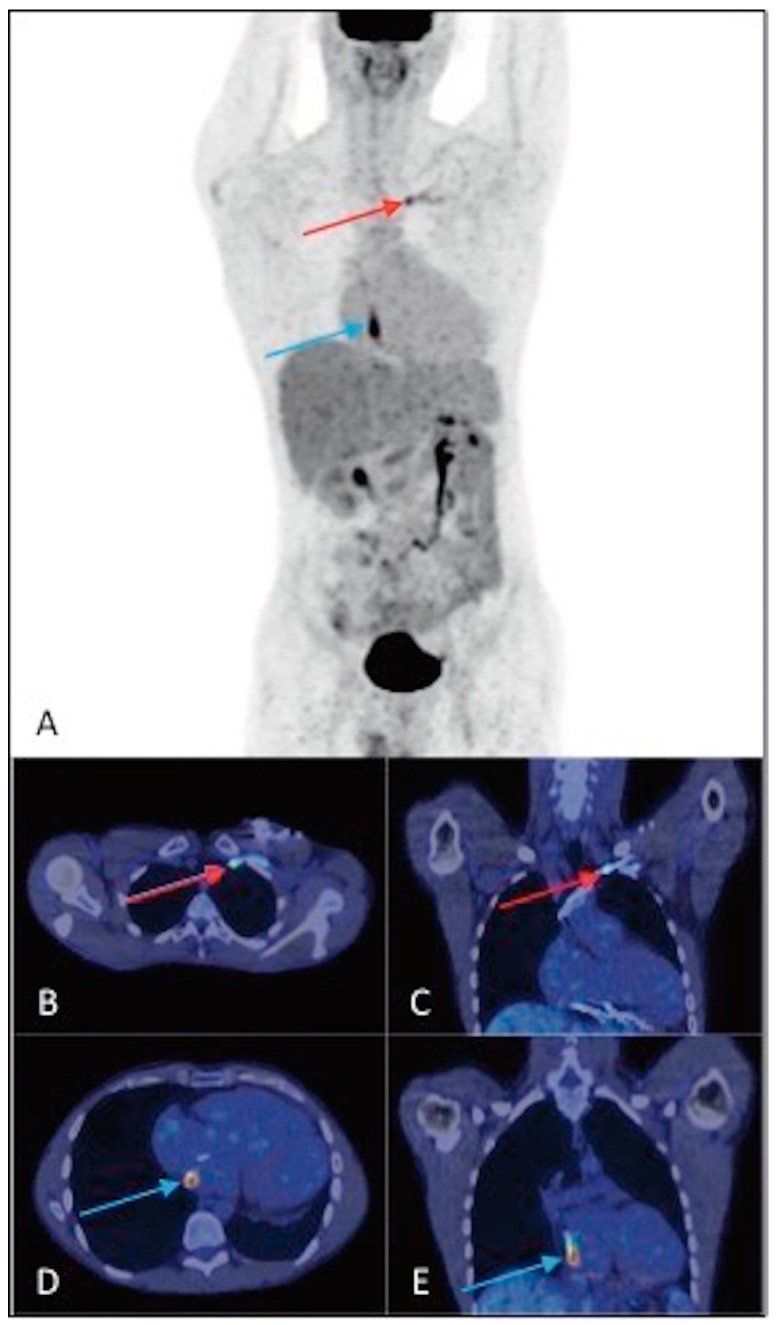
^18^F-FDG PET/CT of a 35-year-old man with implanted ICD, with suspected CIEDI due to persistent alteration of inflammation indices and positive blood culture for *Staphylococcus epidermidis*, in the absence of fever and local signs of infection. The (A) MIP, (B,D) axial fusion and (C,E) coronal fusion images demonstrate the presence of areas of intense and focal increased ^18^F-FDG uptake along the lead pathway, both at the retroclavicular level (SUV_max_ 6.4) (red arrows) and at the intrathoracic level (SUV_max_ 15.6) (blue arrows). The microbiological analysis, following the extraction of the device, confirmed the infection sustained by the pathogen *Staphylococcus epidermidis*.
